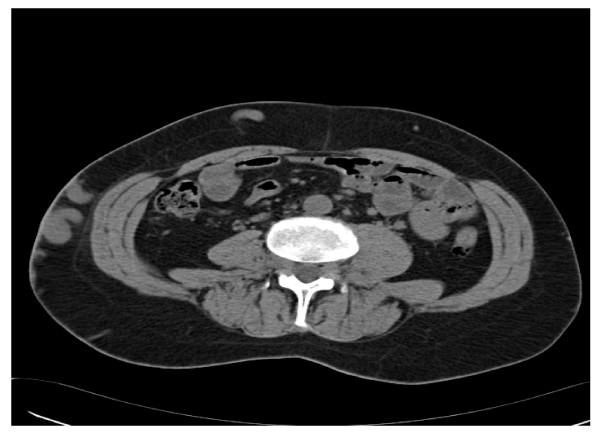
CT abdomen show absent IVC with thrombosis of the very prominent collateral veins in the abdominal wall, corresponding to the right side of the abdomen as seen in figure 1.
